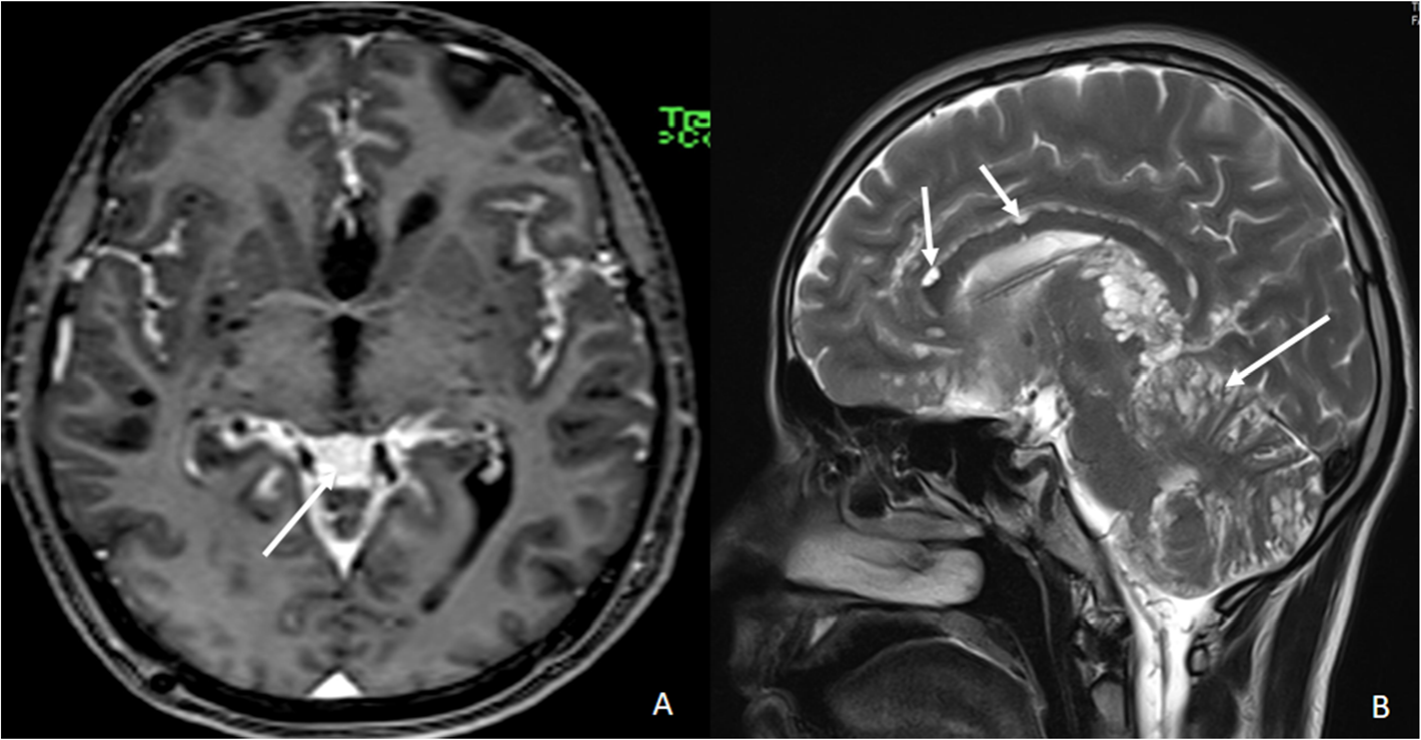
Follow-up magnetic resonance imaging of the brain at our institute 8 months after the onset of the symptoms. A Post-contrast T1W axial image of the brain at the basal ganglia level shows enhancement along the CSF spaces. The white arrow points towards the enhancement along the CSF spaces in the velum interpositum cistern. B T2W sagittal image of the brain in the mid-sagittal plane shows multiple small cysts of varying sizes along the cerebellar folia and the pial surfaces of the corpus callosum. The white arrows point towards the small cysts along the cerebellar folia and the corpus callosum
